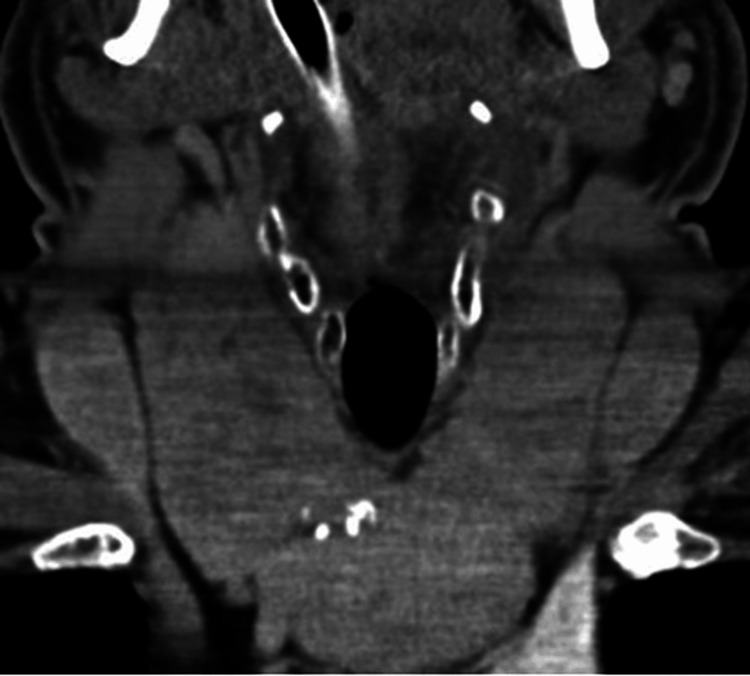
Preoperative contrast CT scan
